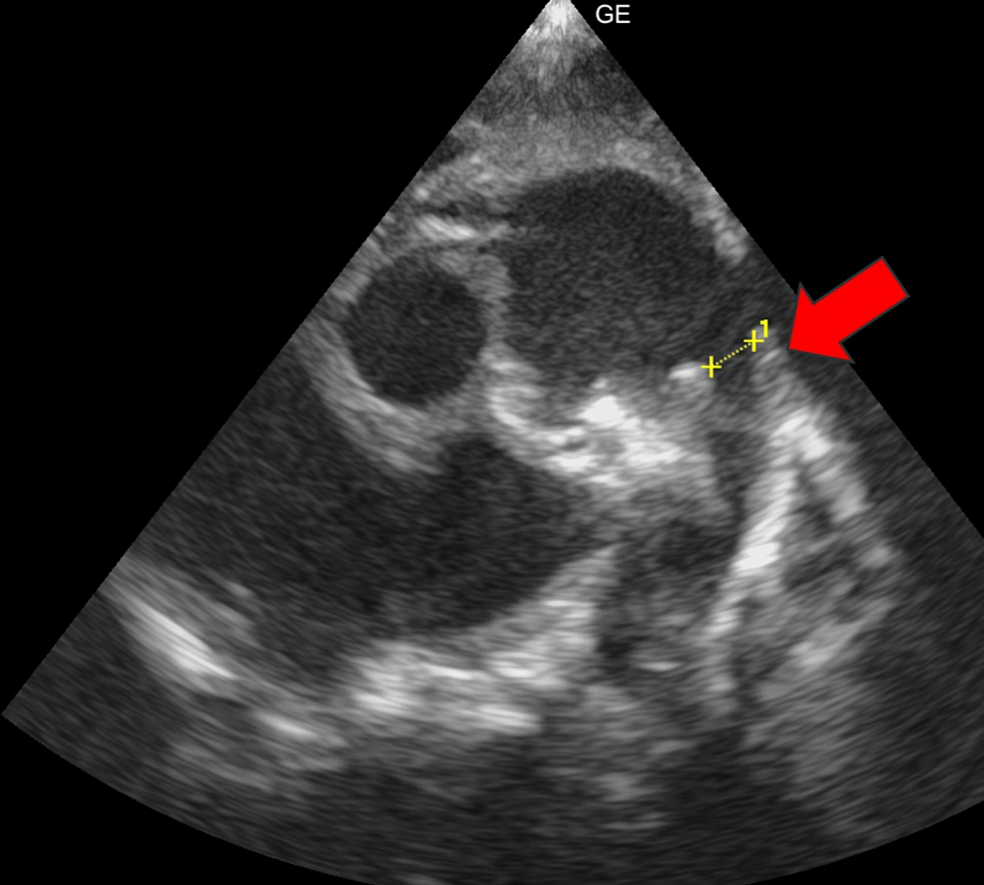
A 2D echocardiogram revealing patent ductus arteriosus (red arrow)
